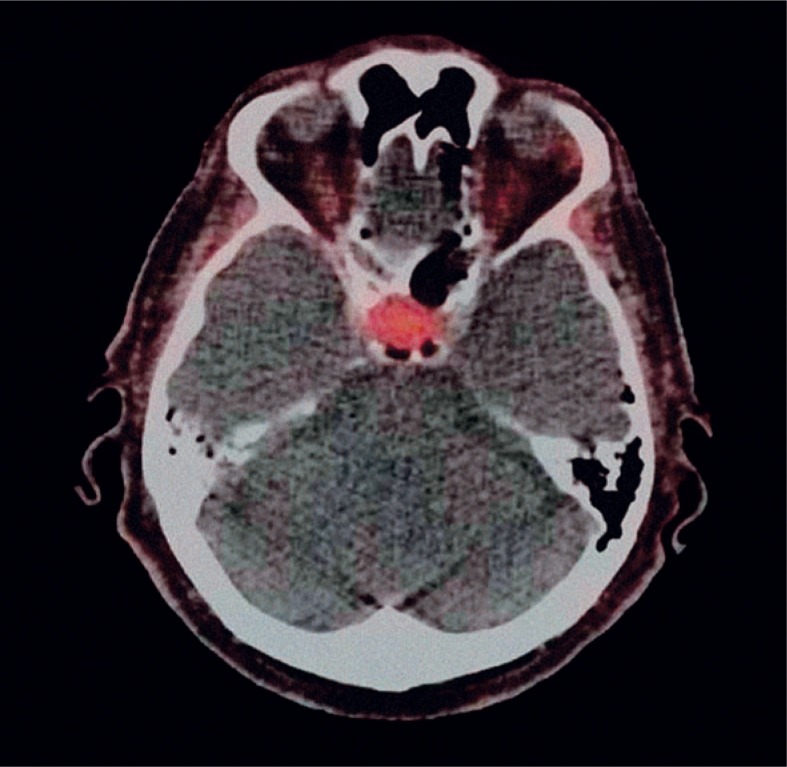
Positron emission tomography-computed tomography axial slice: Macroadenoma of pituitary gland that incidentally took-up ^18^F-fluorocholine (SUVmax 3.7).
